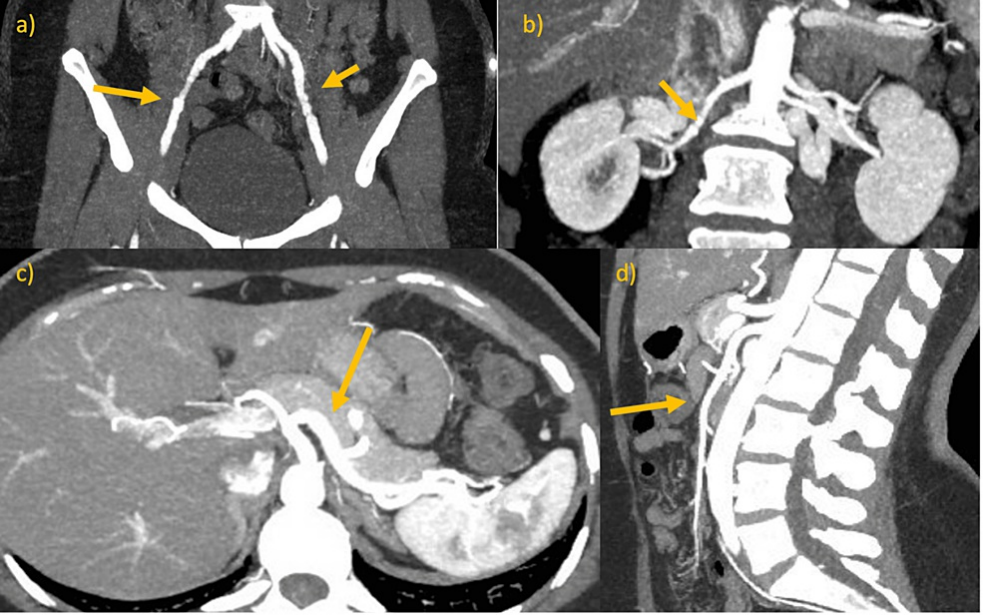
CTA (highlighted by arrows) in coronal plane demonstrating (a) bilateral EIA contour abnormality and (b) mild beading of the right renal artery. (c) Normal coeliac axis in axial plane (highlighted by arrow). (d) Normal superior mesenteric artery in sagittal plane (highlighted by arrow).
